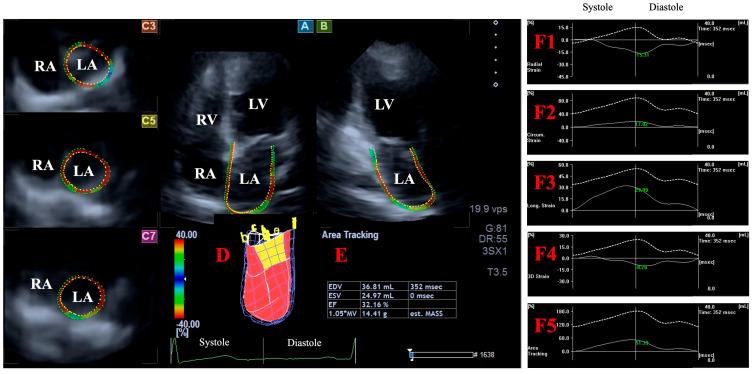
Left atrial (LA) volume and strain assessment by three-dimensional (3D) speckle-tracking echocardiography: apical four-chamber view (A); apical two-chamber view (B); short-axis view at basal (C3), midatrial (C5), and superior left atrial levels (C7) are shown together with a virtual 3D model of the LA (D) and calculated LA volumes (E). Time–global LA radial (F1), circumferential (F2), longitudinal (F3), 3D (F4), and area (F5) strain curves (white lines) and time–LA volume change curve (dashed white lines) are shown as well. LA, left atrium; LV, left ventricle; RA, right atrium; RV, right ventricle; EDV, end-diastolic volume; ESV, end-systolic volume; EF, ejection fraction.
